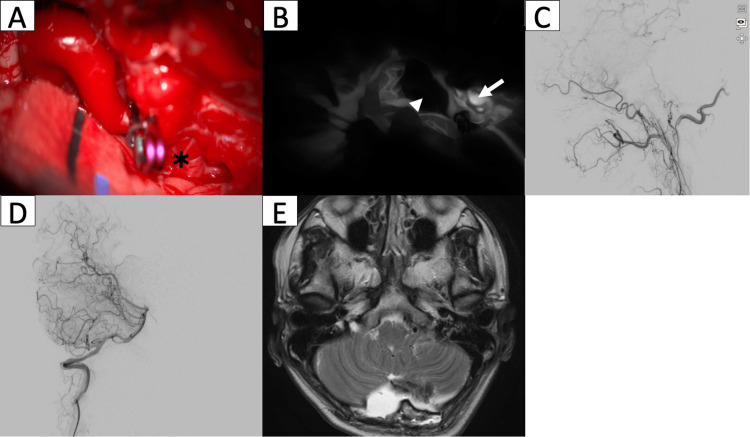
Intraoperative and postoperative imaging Intraoperative image shows a) suboccipital craniotomy with identification and clipping of the main draining vein just distal to the shunt point (asterisk). b) ICG image shows no residual flow on the main draining vein (white arrowhead) with residual flow at the arterial fistula (white arrow). Intraoperative DSA of the external carotid artery (c) and vertebral artery (d) along with postoperative MRI (e) confirmed complete obliteration of the main draining vein with no fistula remaining to be seen. ICG: indocyanine green; DSA: digital subtraction angiography
